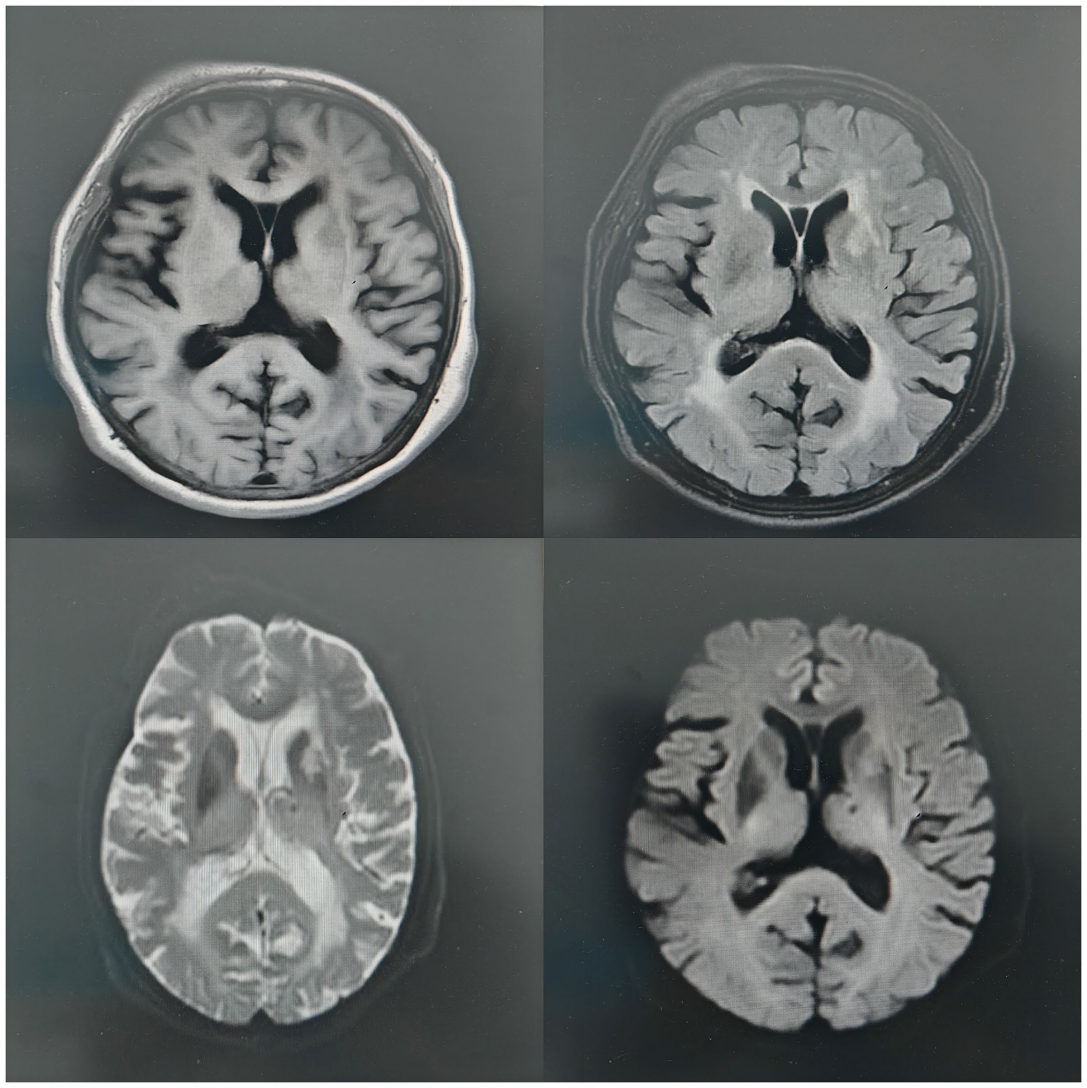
Brain magnetic resonance imaging showed a new cerebral infarction of the left radial crown.
